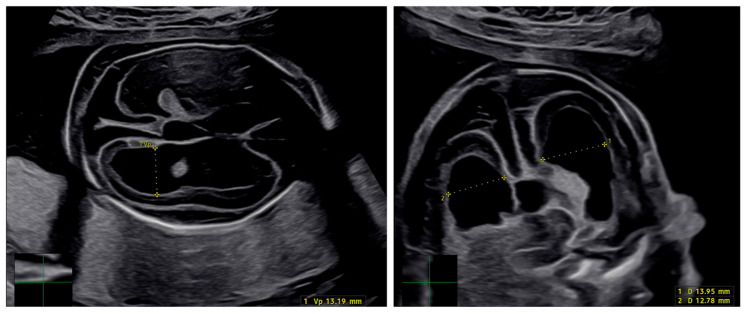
Prenatal ultrasound at 20w + 2d of gestation showing moderate bilateral ventriculomegaly in a fetus with confirmed congenital cytomegalovirus (CMV) infection.
